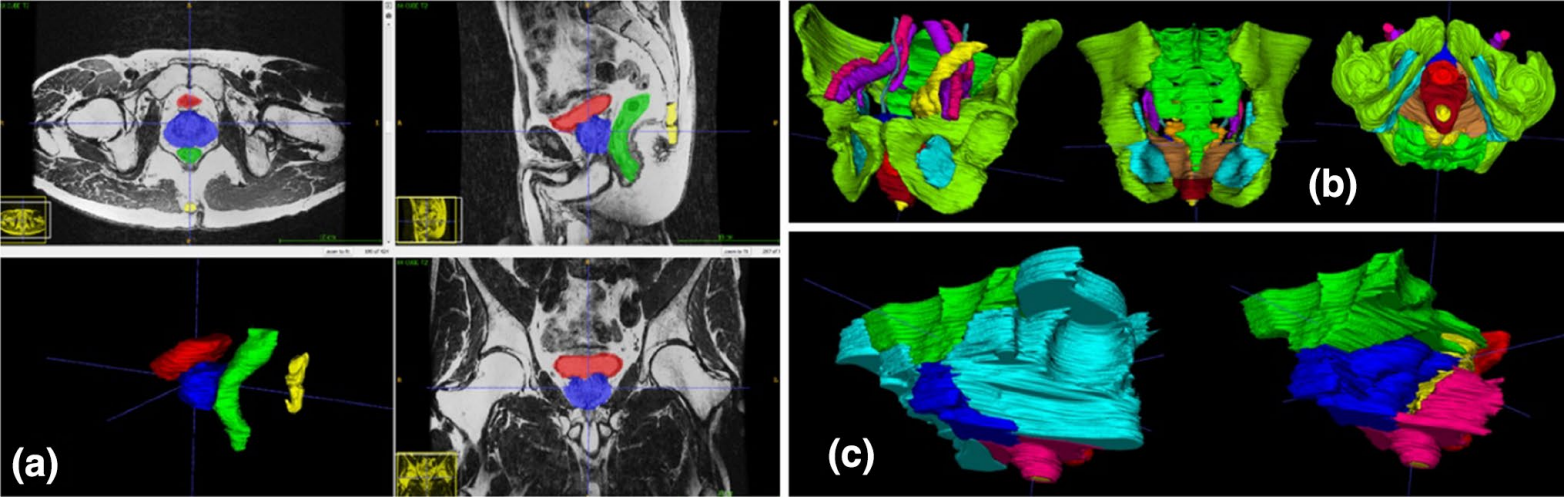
Segmented 3D anatomy and compartments of healthy male volunteer. a Organ delineation in MRI. b 3D anatomy. c 3D compartments with (left) and without (right) lateral compartments
